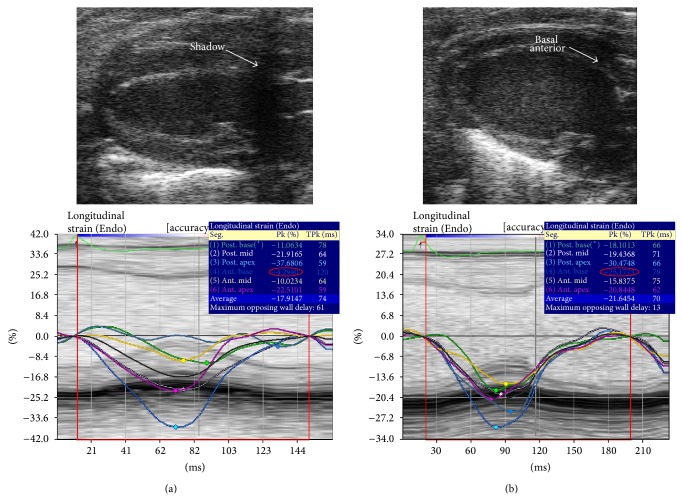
Representation of 2D parasternal long axis where (a) showed basal anterior obscured by shadowing due to sternum but with slight adjustment; shadowing can be eliminated (b). Speckle tracking (below) showed a significant difference in the reading.
